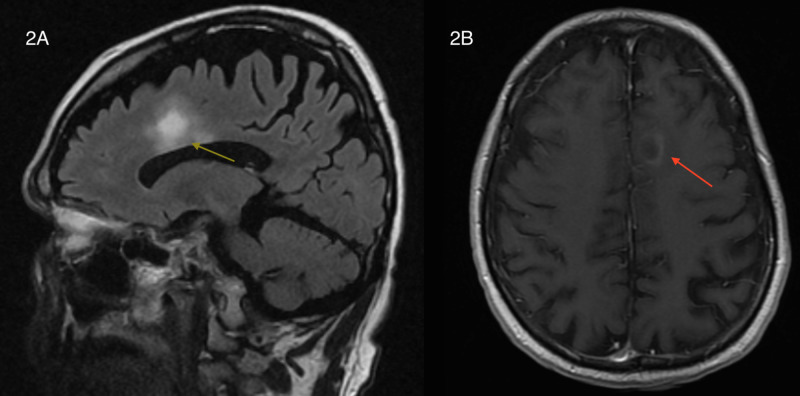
(A) Sagittal FLAIR MRI image demonstrates extension of the large right frontal lobe plaque into the corpus callosum (yellow arrow). (B) T1-weighted axial MRI of the brain with gadolinium enhancement shows peripheral ring enhancement of the right frontal lobe lesion (red arrow). FLAIR, fluid-attenuated inversion recovery
